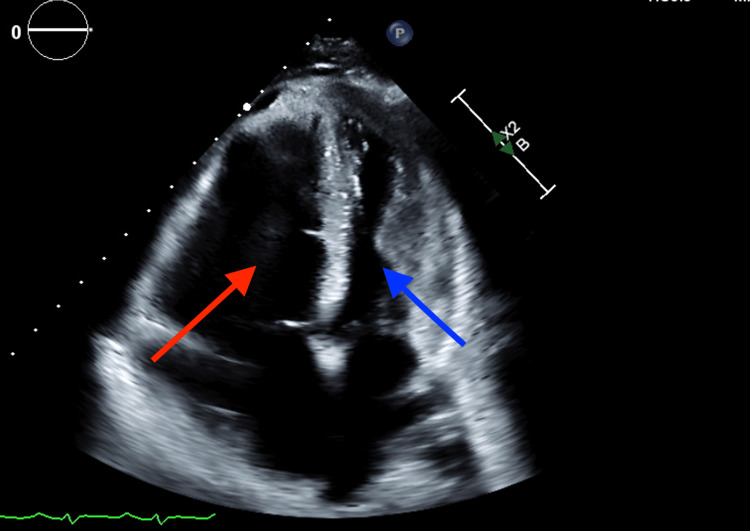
Transthoracic echocardiography showing McConnell's sign with severely dilated right ventricle (red arrow) with free wall hypokinesis and apical sparing and left ventricle (blue arrow).
